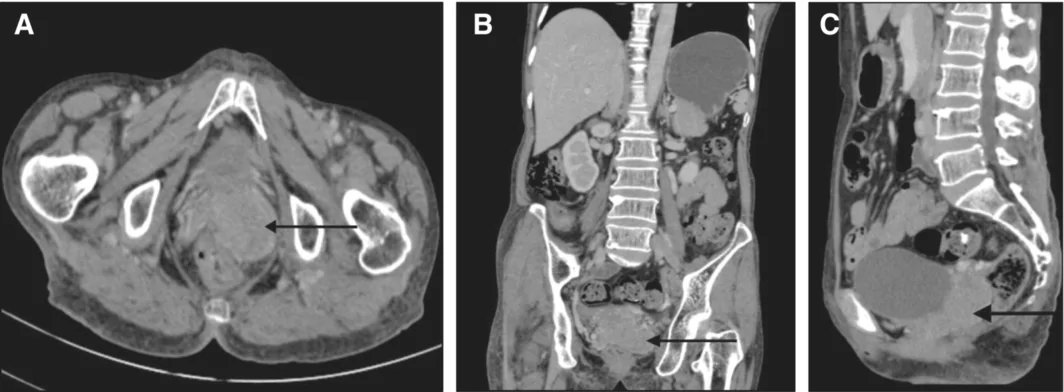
Contrast enhanced computed tomography scan images showing an irregular, ill‐defined, heterogeneous, enhancing soft tissue mass in the uterine cervix. (A) axial section; (B) coronal section and (C) sagittal section.
